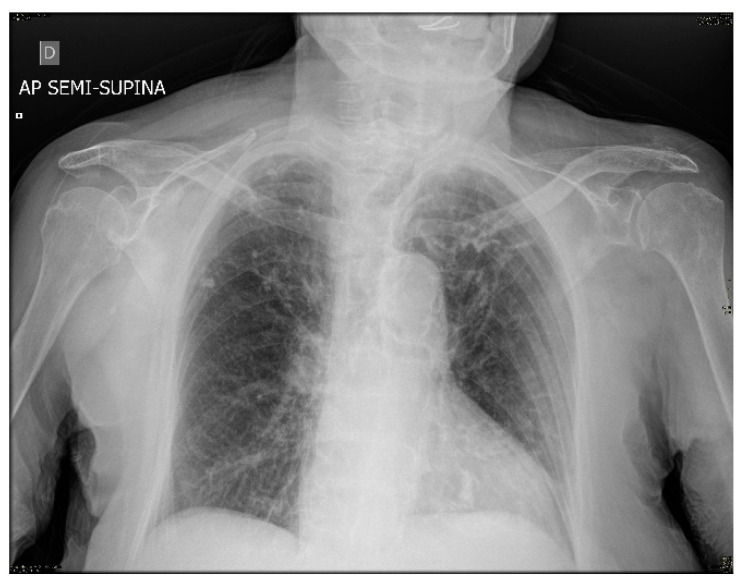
Anterior chest X-ray of the centenarian patient showed a thickening of the peribronchial and bronchilovascular interstitium, probably due to interstitial disease.
